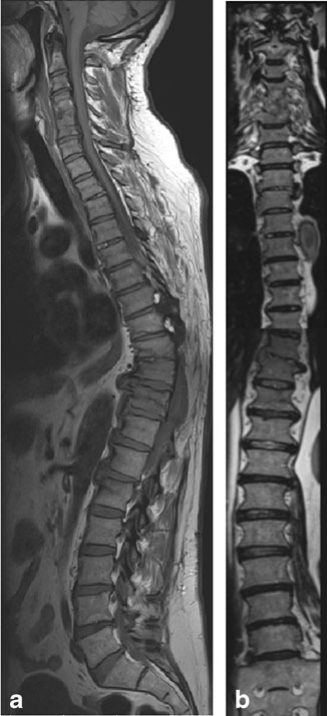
55-year-old man with spinal injury in the past. Sagittal T1-weighted images (a) and coronal reformats of a 3D SPACE sequence (b) demonstrate old injuries, fusion of cervical vertebrae C4 to C6 (most easily appreciated when counting the posterior elements), and one supernumerary vertebra. Additional mobile vertebrae are more common in men than in women
